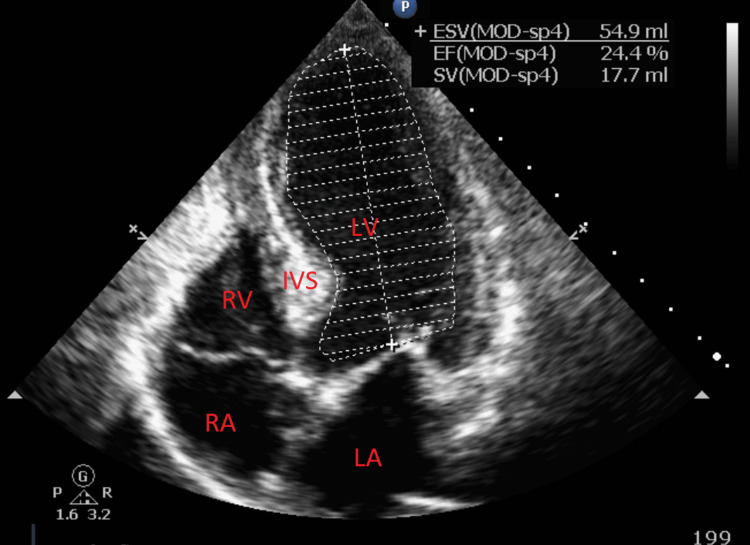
Transthoracic echocardiogram depicting dilated left ventricle with severely reduced ejection fraction. LV-Left Ventricle, LA-Left Atrium, RV-Right Ventricle, RA-Right Atrium, IVS-Intraventricular Septum
